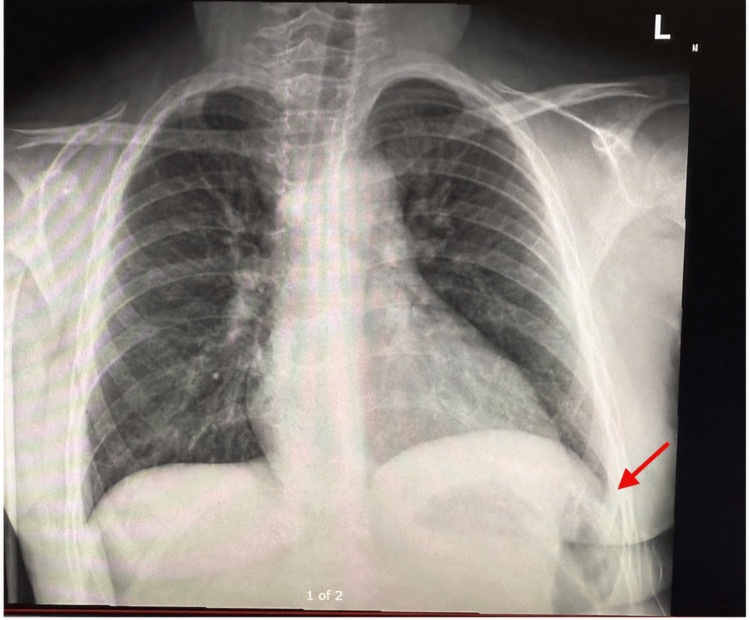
Chest X-ray confirming the incidental small left pleural effusion (red arrow) found on renal ultrasound
